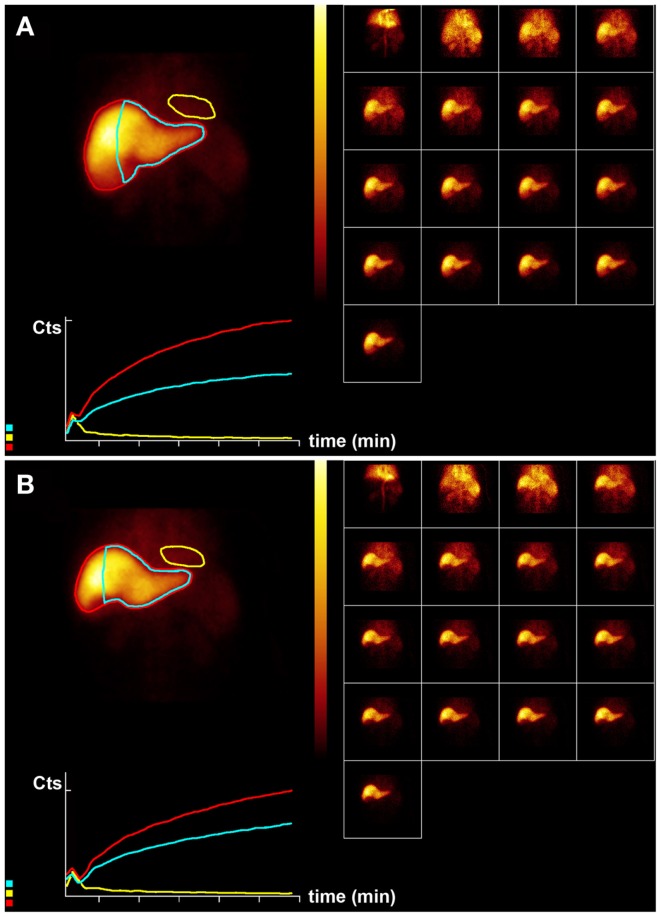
Hepatobiliary scintigraphy before (A) and 6 weeks after (B) right-sided treatment with 1.8 GBq ^90^ Y labeled SIR-Spheres in a 64-year-old male patient with multifocal exclusively right-sided hepatocellular carcinoma with portal vein invasion. Displayed are summed dynamic frames (2 × 10 s/frame), a summed image (20 × 10 s) with regions of interest drawn around the entire liver (red), left liver segments 2–4 (blue), and cardiac blood pool (yellow), and corresponding time–activity curves. The total liver function (body surface area corrected mebrofenin uptake rate) was reduced after treatment from 8.5 to 6.9%/min (red curves). However, function of left liver lobes 2–4 remained unchanged 4.8%/min (blue). This functional increase is only relative and can be explained by lack of functional hypertrophy in the presence of sufficient post treatment functional liver capacity.
